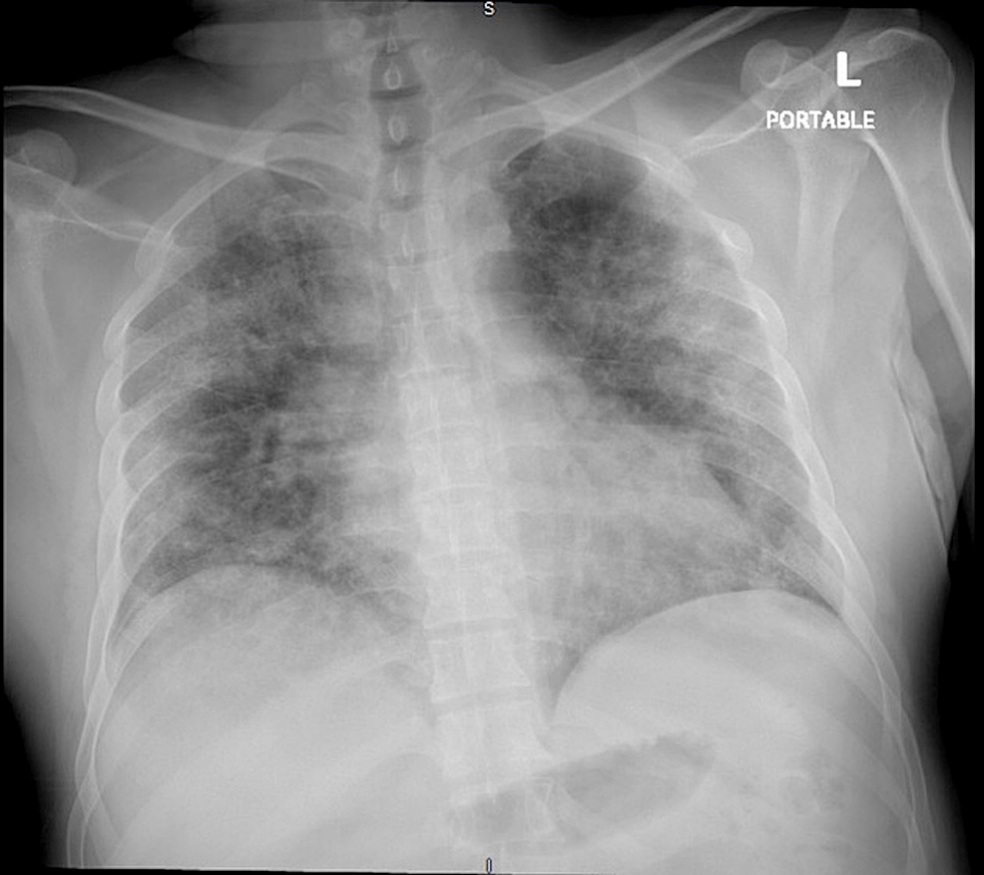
CXR showing bilateral reticular opacities consistent with multifocal pneumonia CXR, chest X-ray
